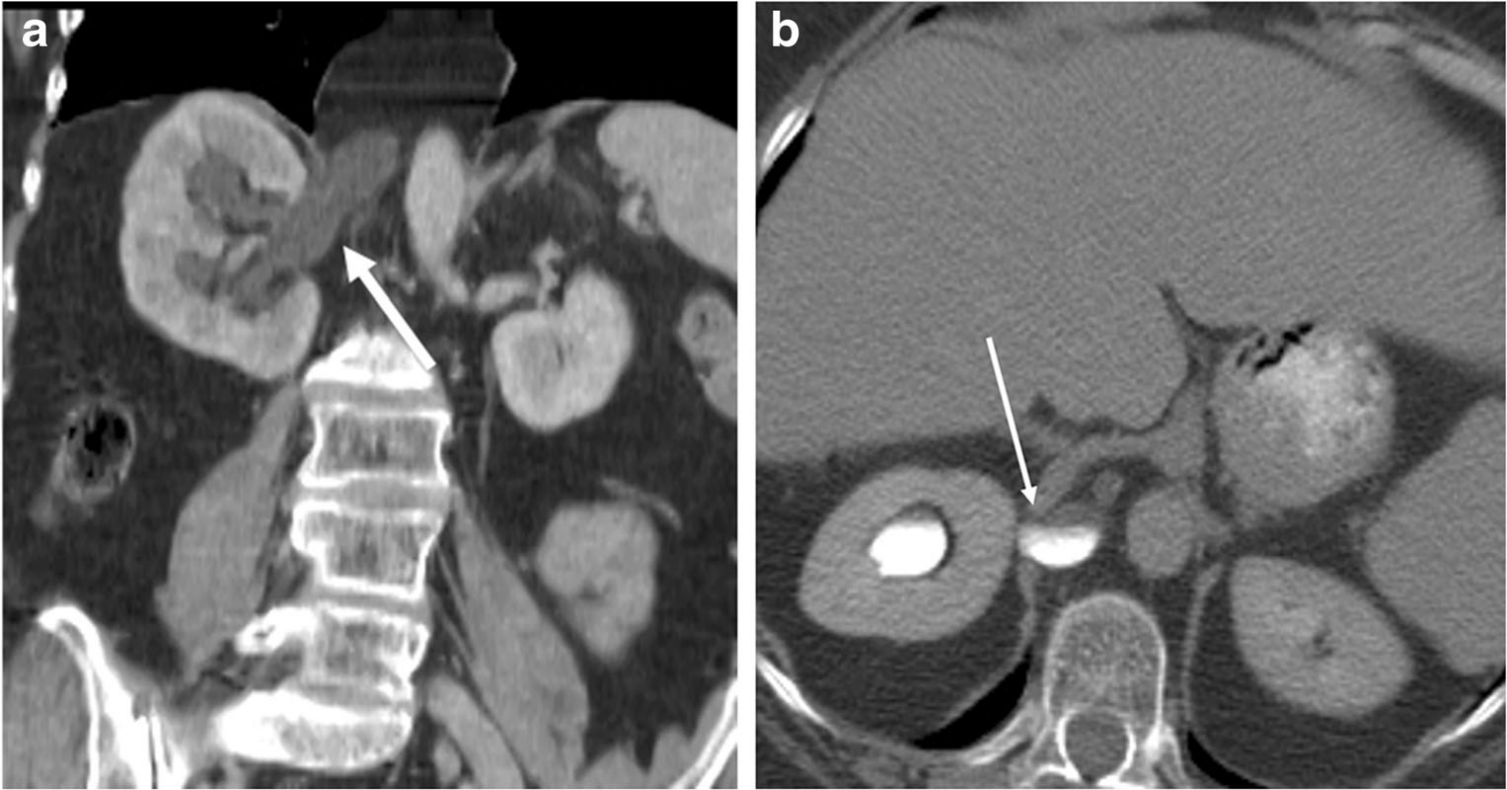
a, b Coronal (a) venous phase and axial delayed excretory phase (b) CT from a patient with hydroneprosis identifies a focal defect in the right posterior diaphragm (white arrow) with herniation of right ureter into the thorax. There is ureteric obstruction at the neck of the hernia with resultant hydronephrosis
